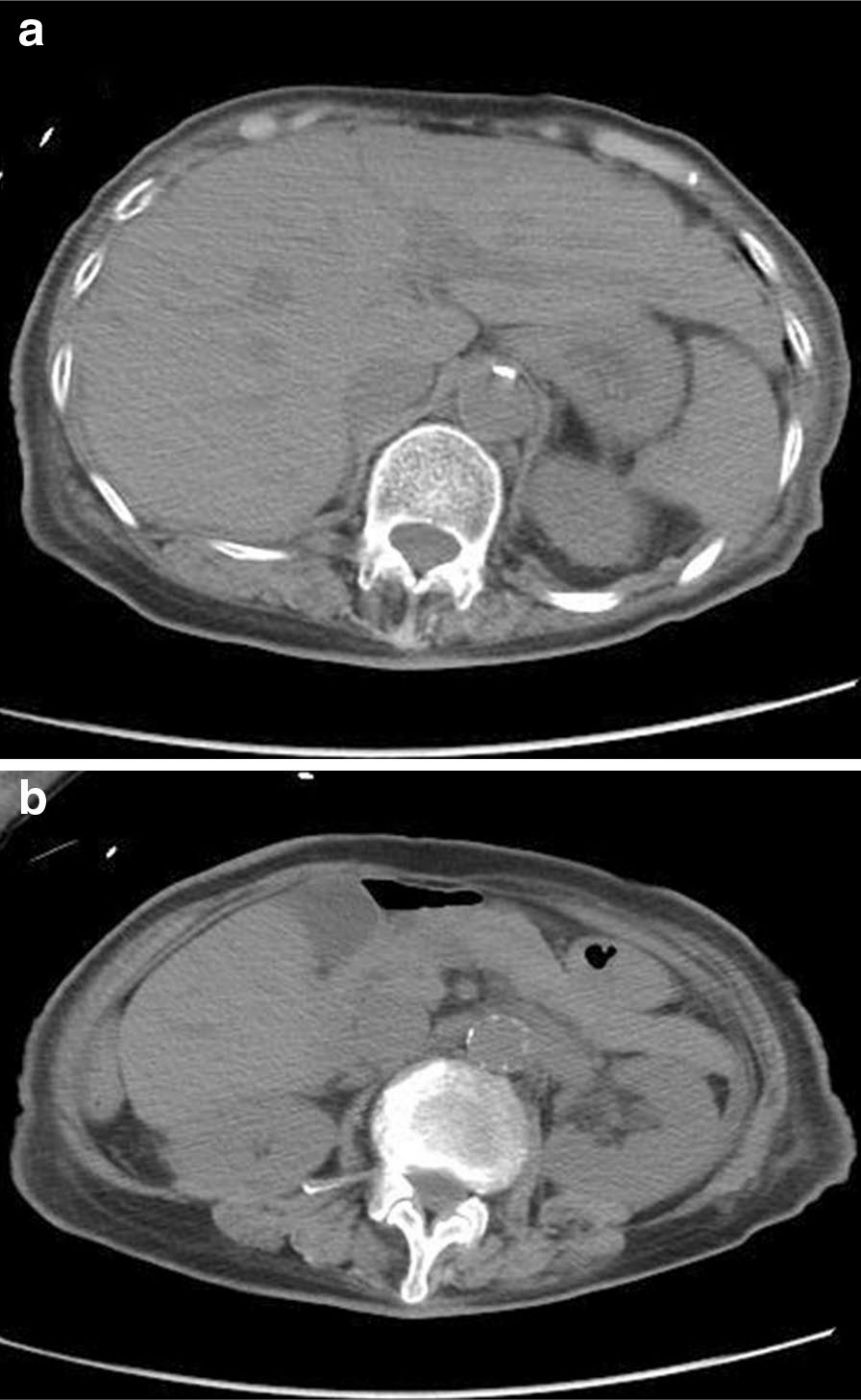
Axial non-contrast computed tomography at the level of the left portal vein of the liver (a) and pancreatic body (b). No abnormal findings were observed.
